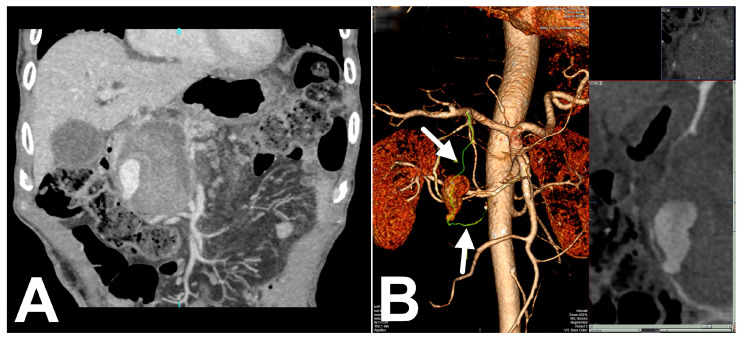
(A) Coronal section contrast-enhanced abdominal computed tomography (CT) demonstrates central enhancing component in previously verified walled-off pancreatic necrosis in the head of the pancreas. (B) 3D Volume Rendering CT shows central enhancing component surrounded with necrotic tissue and nearly formed hematoma that indicates pseudoaneurysm originating from the posterior inferior pancreaticoduodenal artery.
